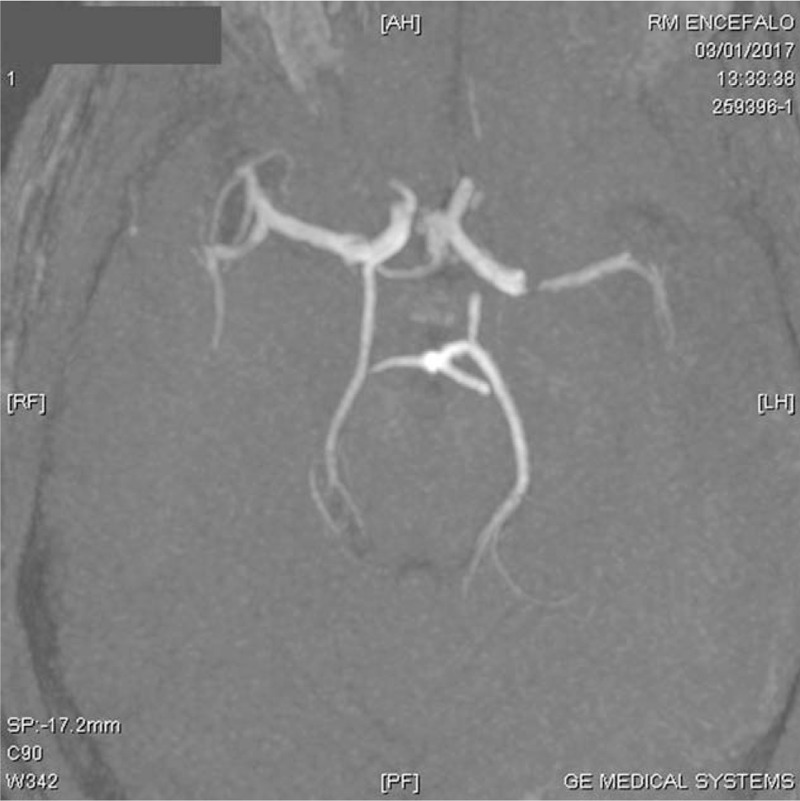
Angio MRI shows a filling defect of left middle cerebral artery.
